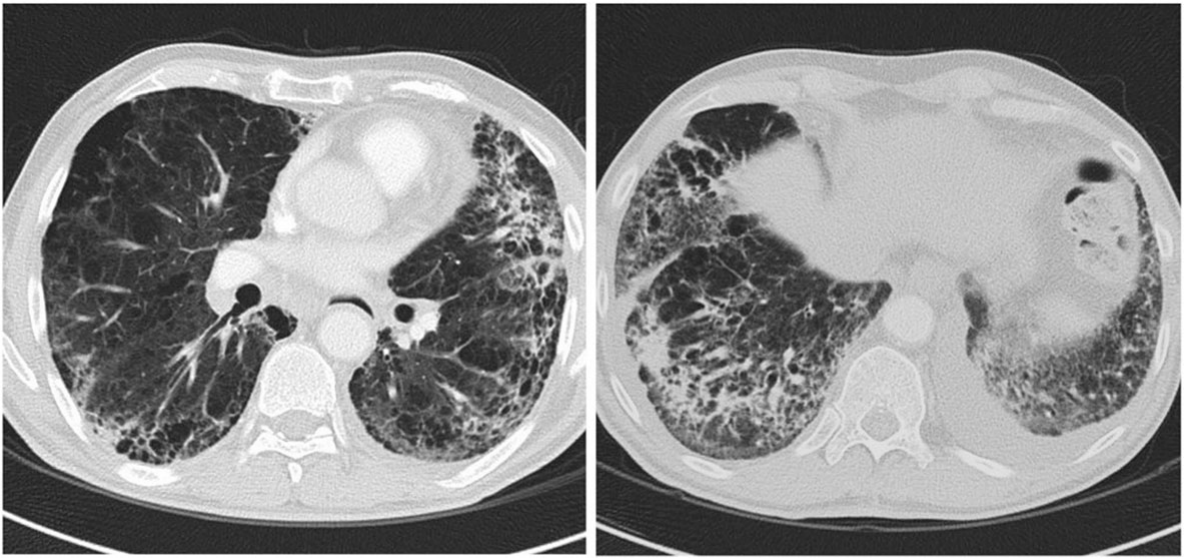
Chest CT scan image obtained at the time of clinical presentation with respiratory symptoms reveals nonspecific diffuse alveolar involvement
